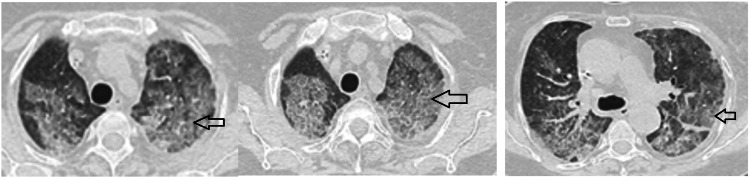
A 67-year-old female patient's CT scan reveals diffuse ground-glass regions through the center and periphery of both lung hemifields Arrows: ground-glass opacities CT: computed tomography
